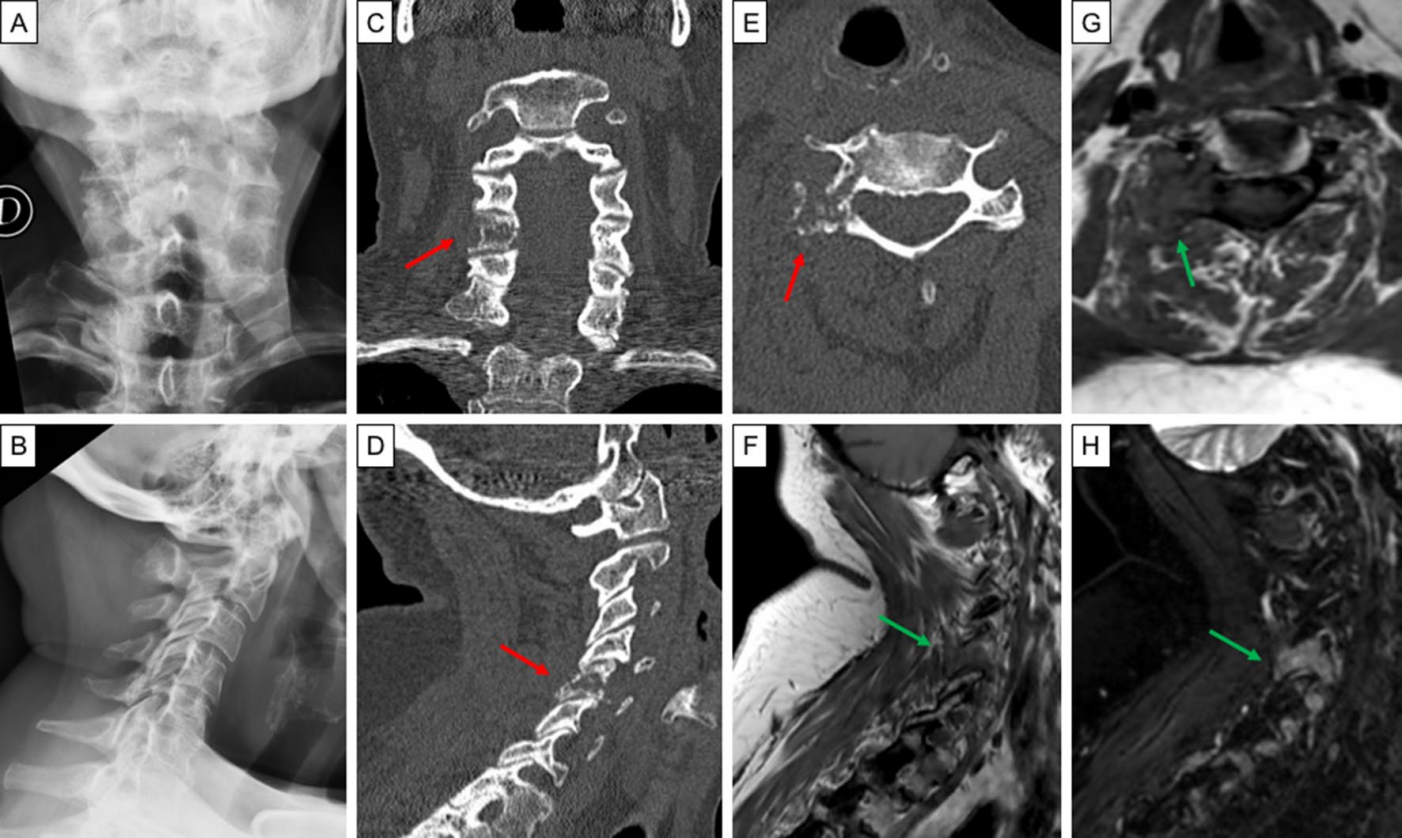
Images from a 55-year-old female with poorly differentiated thyroid cancer and cervical pain. The patient underwent cervical spine plain radiograph (a postero-anterior view, b latero-lateral view) that was reported as negative. Therefore, after a few days, she underwent cervical spine CT. The CT multiplanar reconstructions images (c axial view, d coronal view, e sagittal view) showed the presence of an osteolytic bone lesion involving the peduncle and the zygapophyseal joint with minimal involvement of the hemisoma of the fifth cervical vertebra (red arrow). The MR images (f T1-weighted sagittal view; g T1-weighted axial view; h T2-weighted with fat suppression sagittal view) confirmed the CT scan findings, with a lesion which resulted hypointense in T1 and hypertense in T2 (green arrow). *CT* computed tomography, *MR* magnetic resonance
